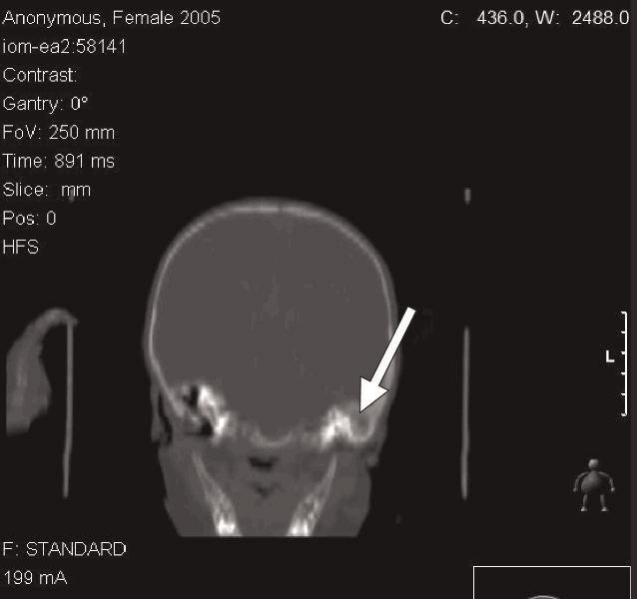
Coronal computed tomography scan of the head and neck taken at time of presentation using bone window settings, showing mastoid abscess on the left side.
